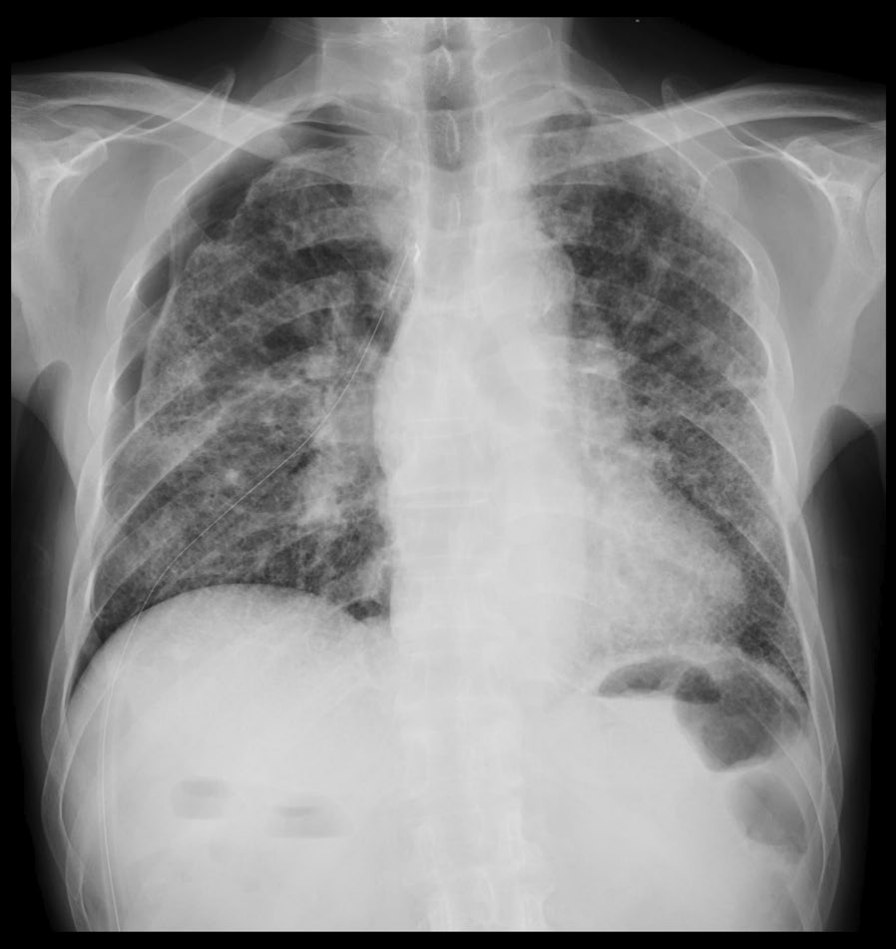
Preoperative chest X-ray showing incomplete expansion of the right lung
